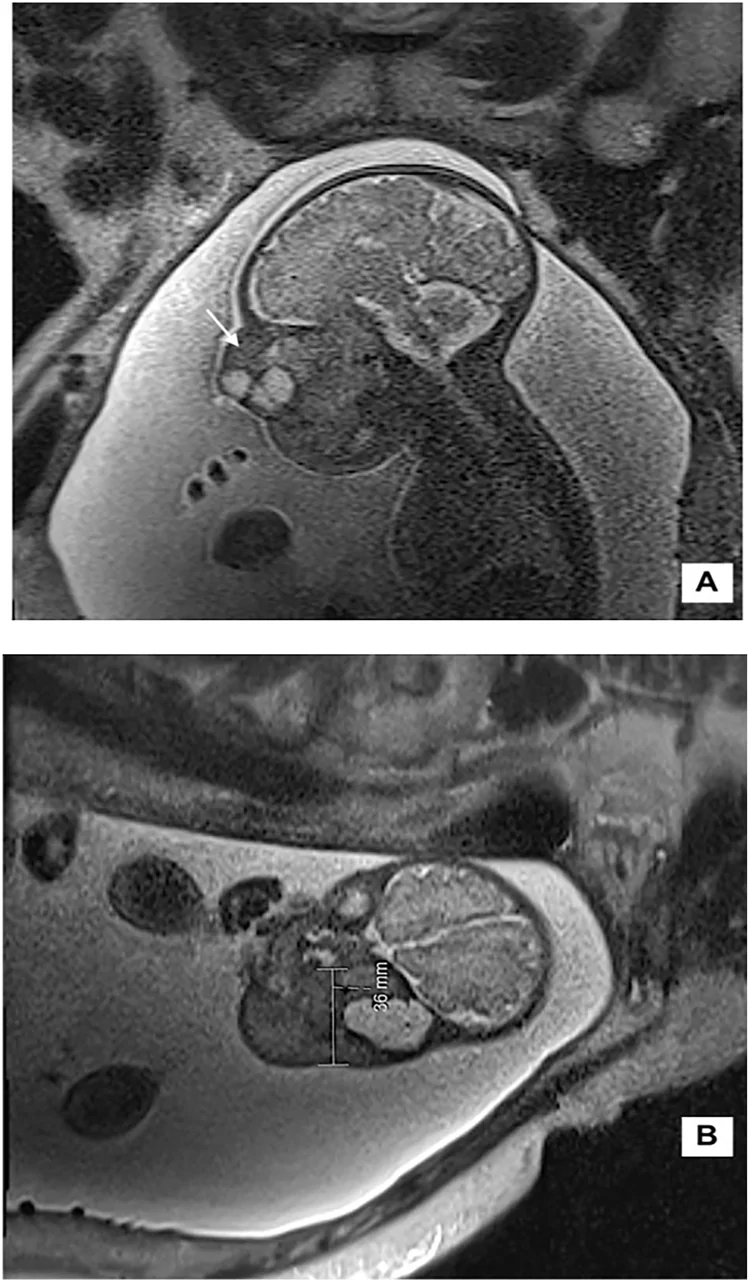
Fetal magnetic resonance imaging findings. (A) Mass (indicated by the arrow) located in the left retroorbital space, pushing the globe anteriorly, with a solid-cystic internal structure. (B) Sagittal section showing the lesion’s extension into the retropharyngeal space and its approximately 6 cm craniocaudal dimension.
